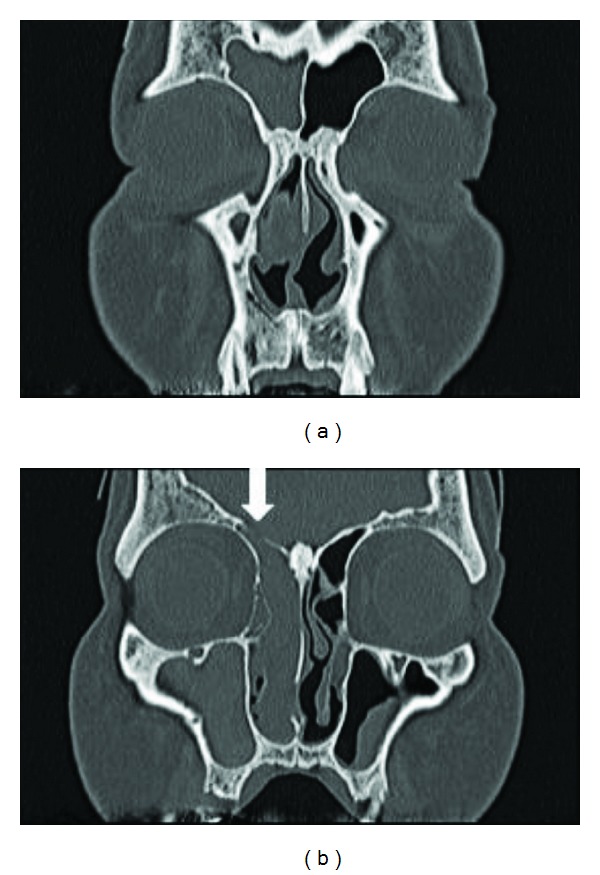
CT showed an isodense mass which occupied the right nasal cavity, maxillary, ethmoid, and frontal sinuses, with no erosion of the bony walls. ↓: A small defect in the cribriform plate.
